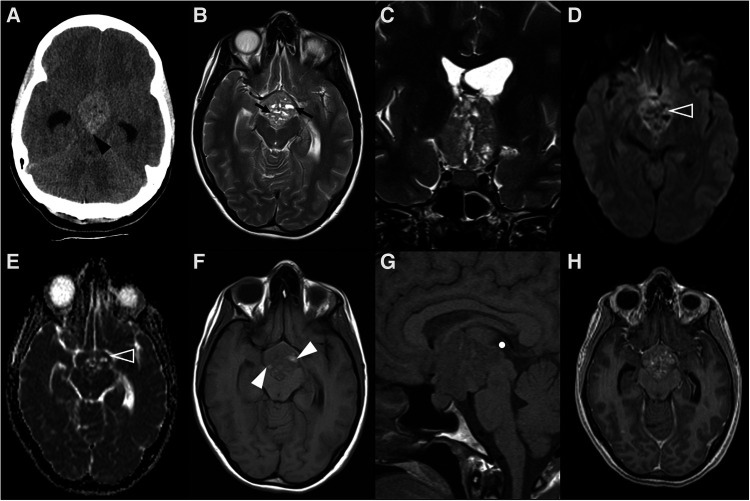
Choriocarcinoma in a 17-year-old female presenting with central diabetes insipidus. Axial head CT scan (A) reveals a spontaneously hyperdense mass centered in the supra-sellar cistern and with extension towards the interpeduncular cistern (black arrowhead). Brain MRI imaging including axial (B) and coronal (C) T2WI, DWI (D) and ADC map (E), as well as axial (F) and sagittal (G) T1WI, better depicts a polilobulated lesion, which is well demarcated from the pituitary gland. It shows heterogeneous signal, including levels (black arrows) and areas of spontaneous T1 hyperintensity (white arrowheads) in keeping with hemorrhagic/proteinaceous content, and foci of restricted diffusion (empty white arrowheads). The spontaneous T1 bright spot of the neurohypophysis cannot be detected on sagittal pre-gadolinium T1WI (G). On post-gadolinium axial, heterogenous enhancement is observed. Note the almost complete obliteration of the third ventricle (C and white circle in G), the optic chiasm and tracts (white arrows in C), and the cerebral peduncles (black arrowheads in H), as well as mild hydrocephalus (asterisks in A,C).
